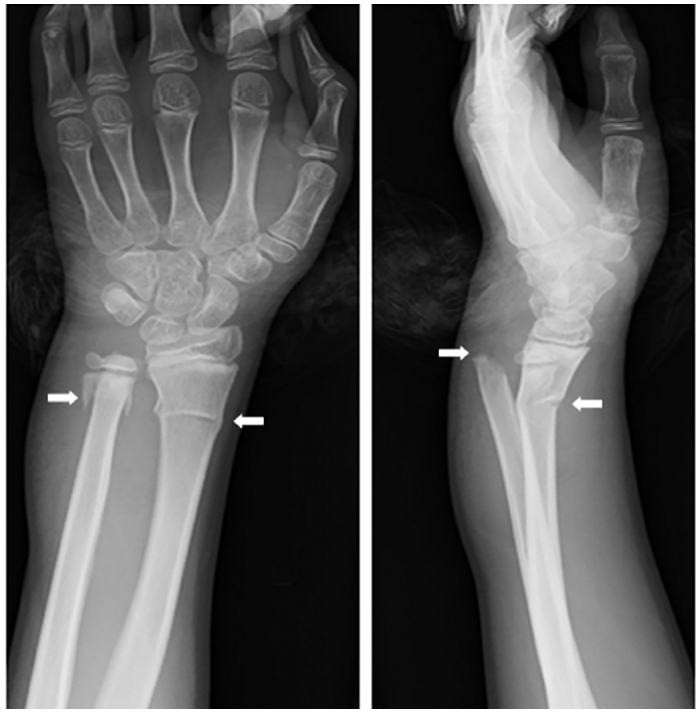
Anteroposterior (left) and lateral (right) radiographs of the distal forearm and wrist joint. Radiographs show the radius and ulnar fractures (arrows).
